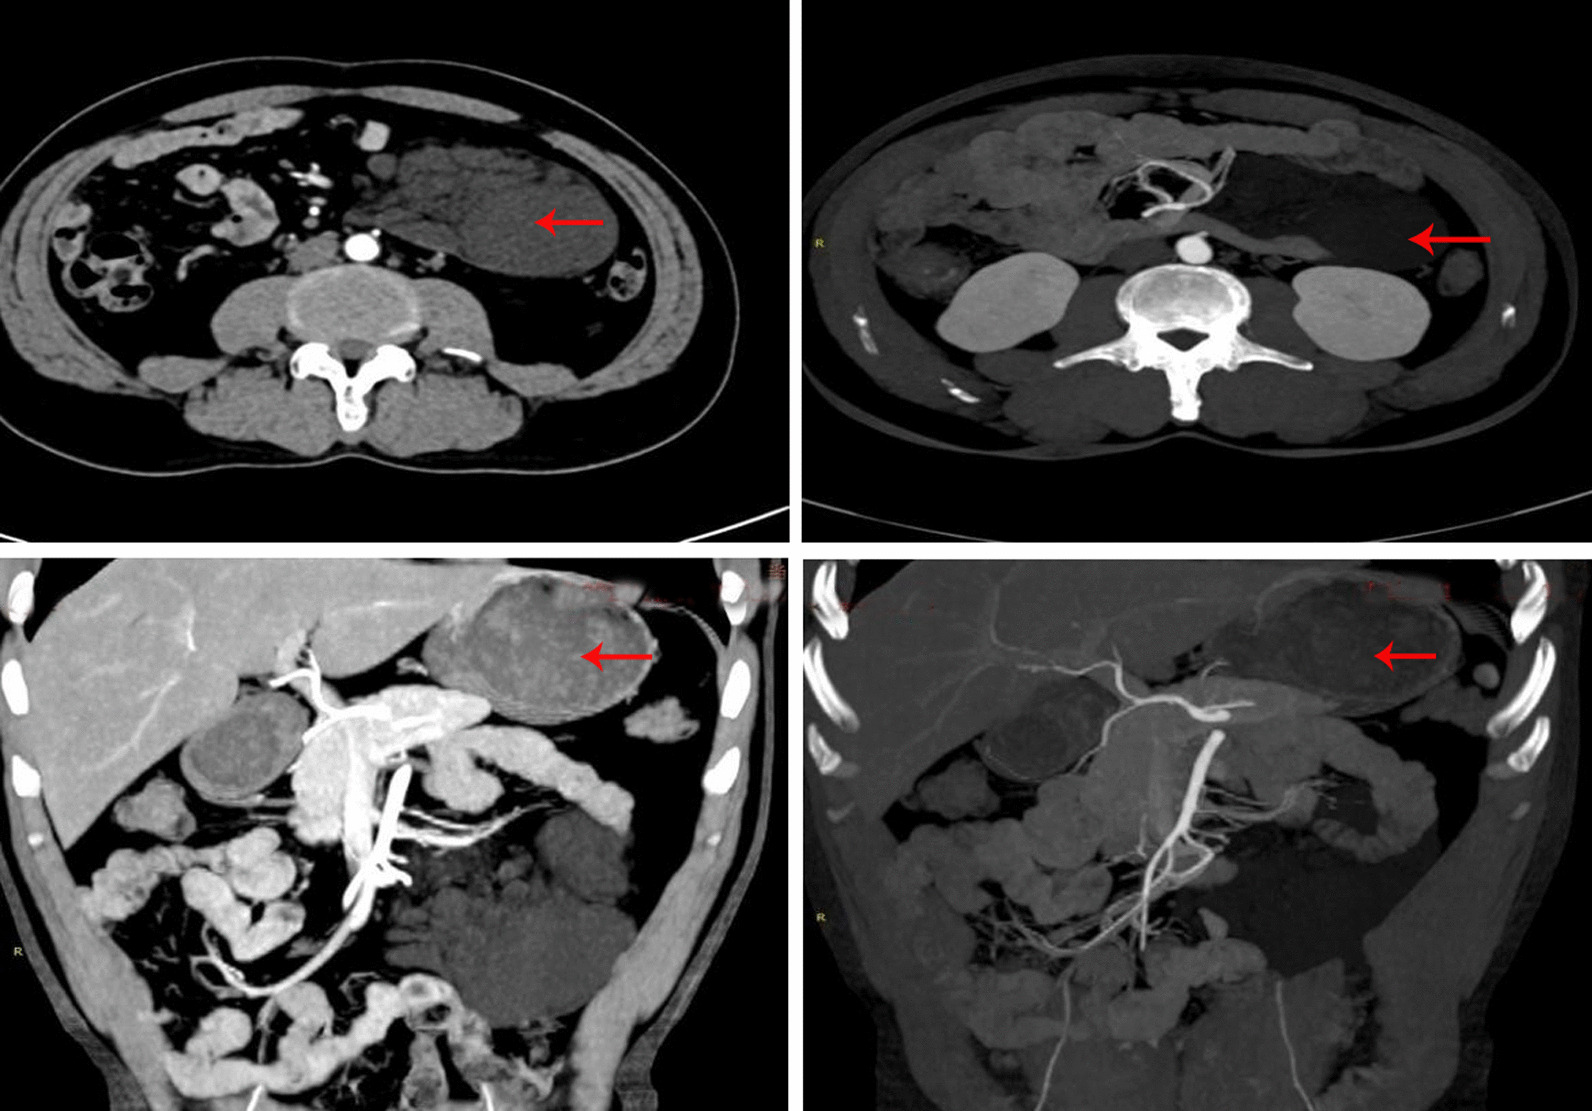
Abdominal enhancement CT revealing an irregular mass (arrows) originating from the mesentery in the left mid-abdomen. The maximum cross-sectional area of the mass was approximately 5.5 cm × 10.6 cm, and a low-density shadow of the fat inside can be observed. The contrast-enhanced CT scans show no obvious enhancement. The mass constricted the adjacent small bowel and pushed it out (Case 1)
